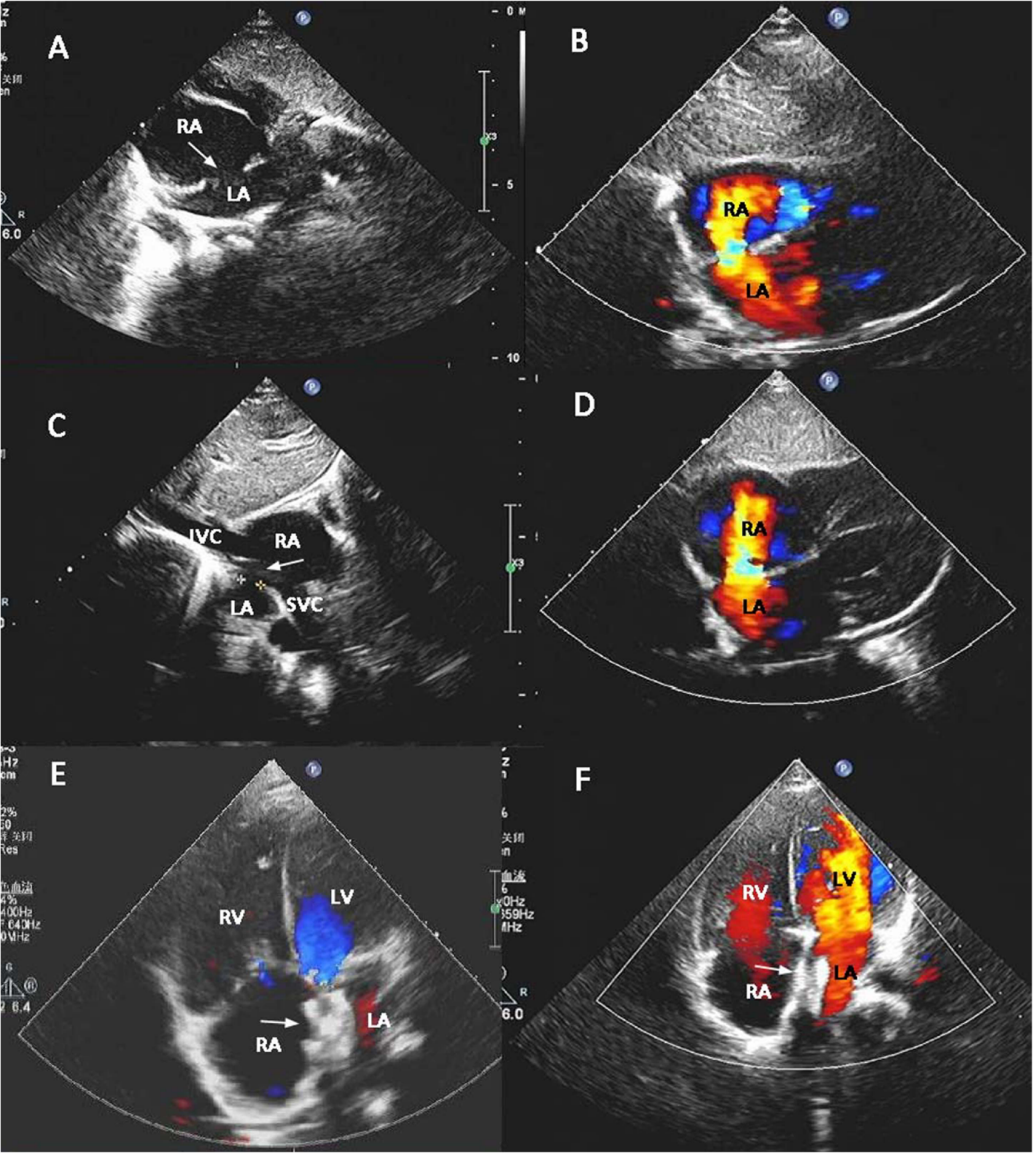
a Preoperative echocardiography shows the ASD, the arrow showing the defect; b The residual shunt 1 month after initial surgery. The red circle indicates the deficient posteroinferior rim; c Bicaval view of the residual shunt 3 months after initial surgery, the arrow showing the defect with minimum rim in the posteroinferior aspect; d The residual shunt 1 year after initial surgery; e Device closure of the residual shunt, the arrow showing the occluder. LA = left atrium; LV = left ventricle; f Three months after device closure of the residual shunt, the arrow showing the occluder. LA = left atrium, LV = left ventricle, A = right atrium, RV = right ventricle, IVC = posteroinferior vena cava, SVC = superior vena cava
